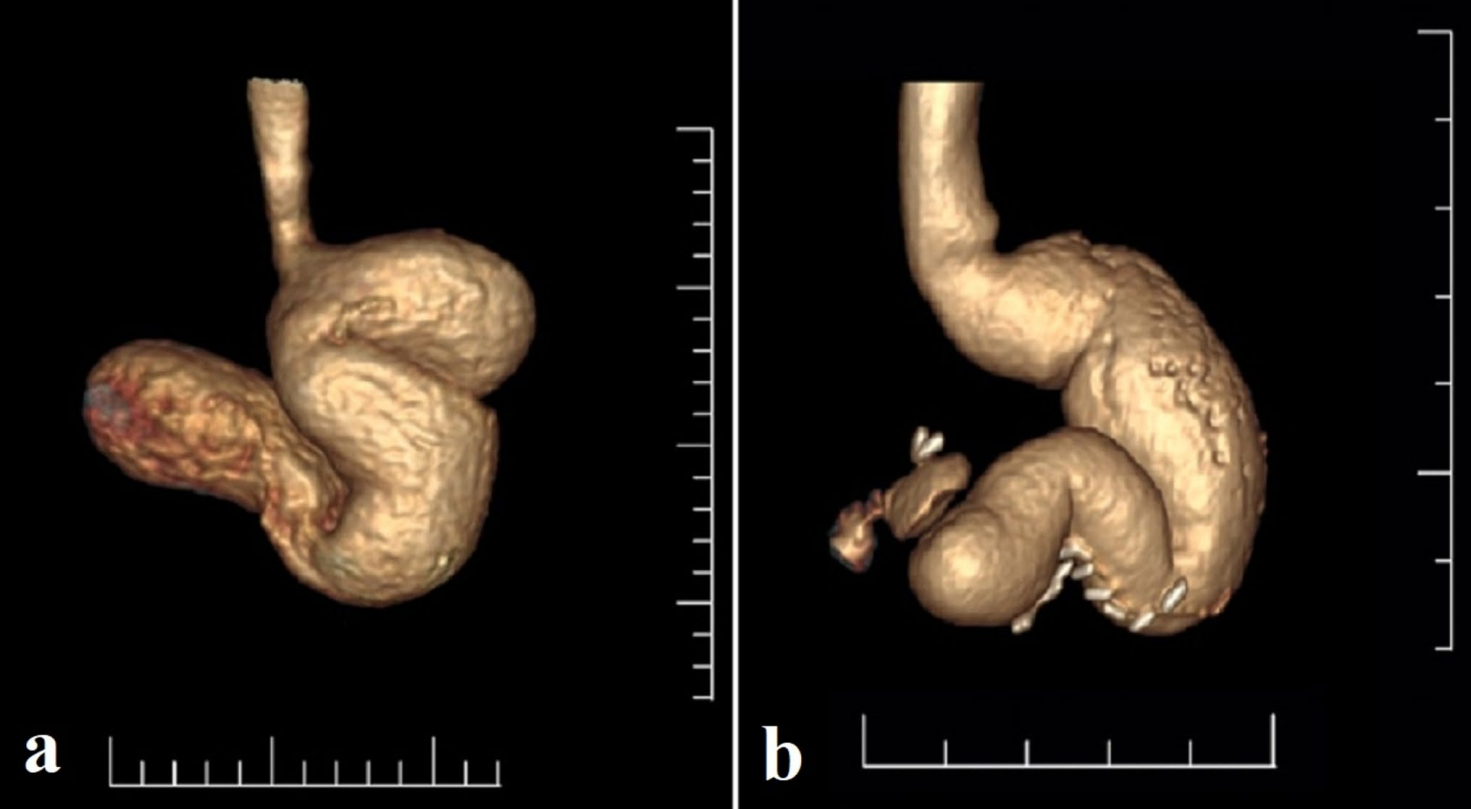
CT gastric volumetry of two cases showing: a GT with primary dilation, b GT with primary diffuse dilation
